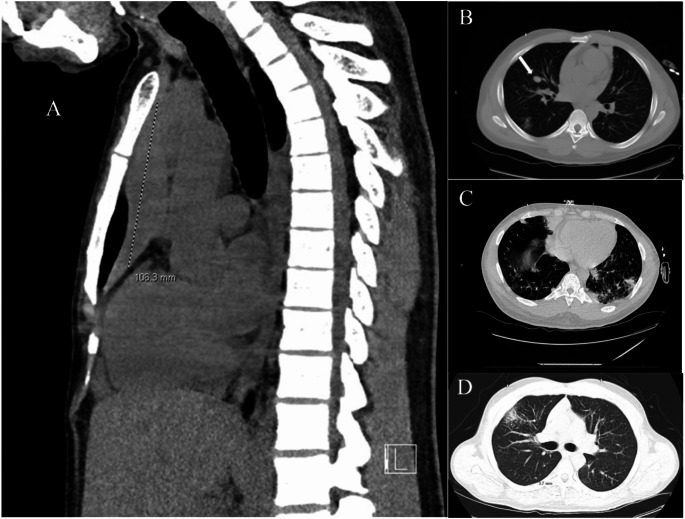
CT Chest at presentation. (A) A 4.7 × 3.0 × 10 cm homogeneous soft tissue density lesion in the anterior mediastinum; (B) A 1.8 cm nodule in the right middle lung lobe (white arrow); (C and D) Focal, patchy opacities
